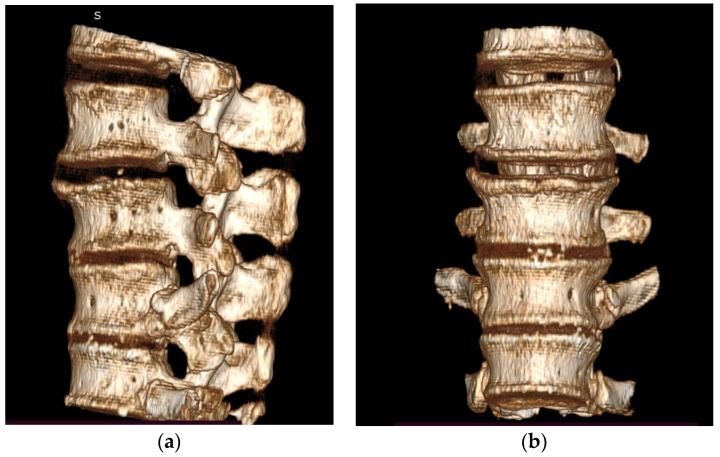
(a) CT image 3D reconstruction of the analyzed spine segment–lateral view; (b) anteroposterior view.
